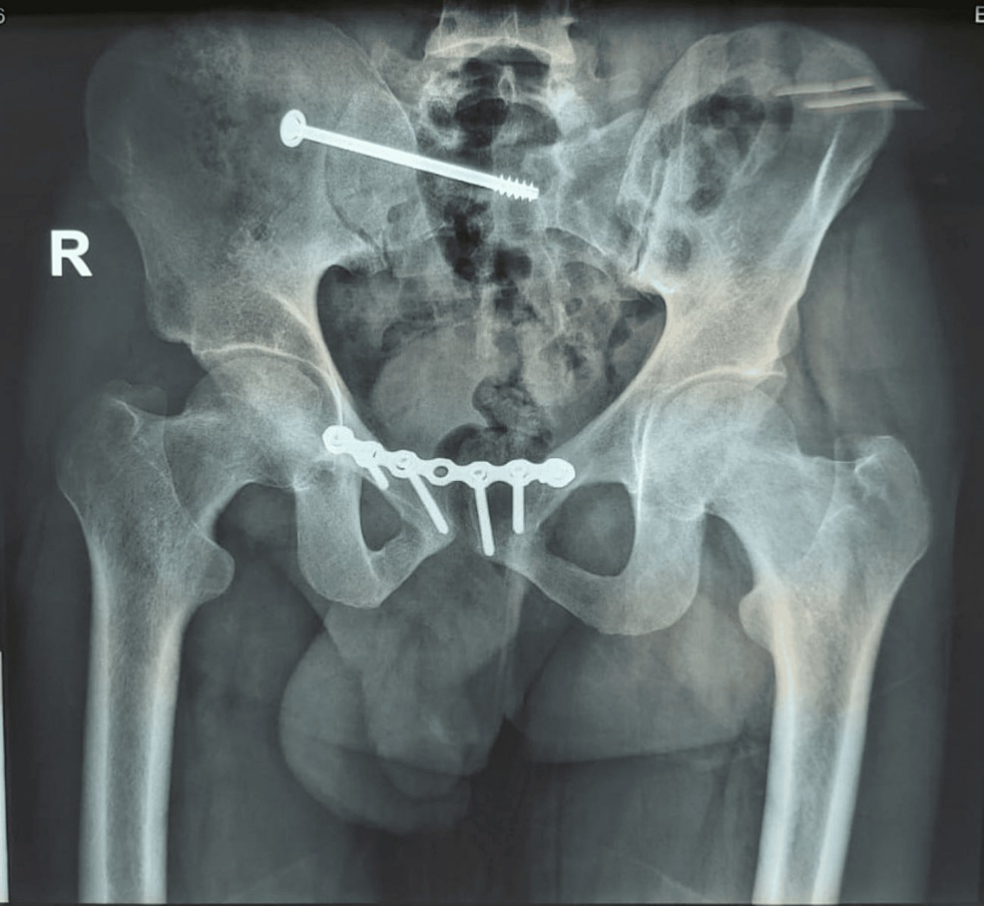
One-year follow-up X-ray posteroanterior view of the pelvis showing complete healing at the sacral fracture fixed with iliosacral screw with stabilization of anterior pelvic ring by symphysial plating Image Credit: First Author
